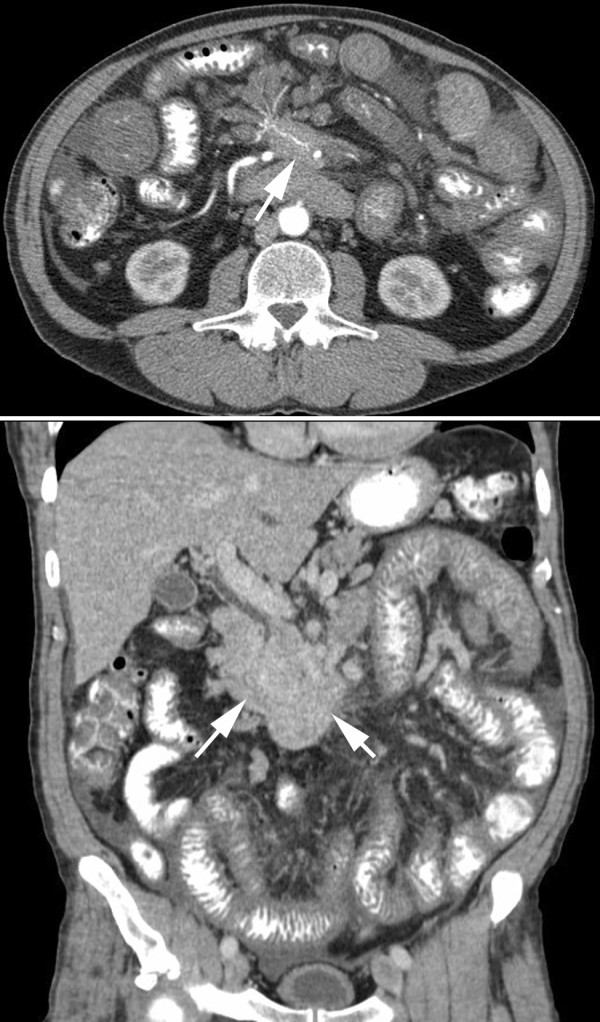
Preoperative abdominal CT scan demonstrating large mesenteric soft tissue mass (white arrows) encasing the mesenteric vasculature with evident bowel wall and mesenteric edema, along with trace ascites fluid.
